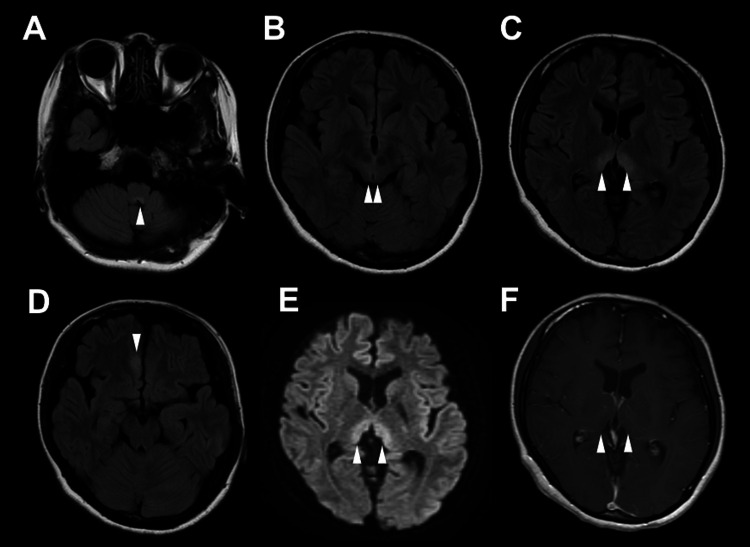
Magnetic resonance imaging findings Fluid-attenuated inversion recovery images show high-signal lesions in the bilateral area postrema (arrowhead in A), bilateral periaqueductal gray matter (arrowheads in B), bilateral medial thalamus (arrowheads in C), and medial part of the right frontal lobe (arrowhead in D). Diffusion-weighted imaging shows high-signal lesions in the bilateral medial thalamus (arrowheads in E), although no contrast enhancement (arrowhead in F).
